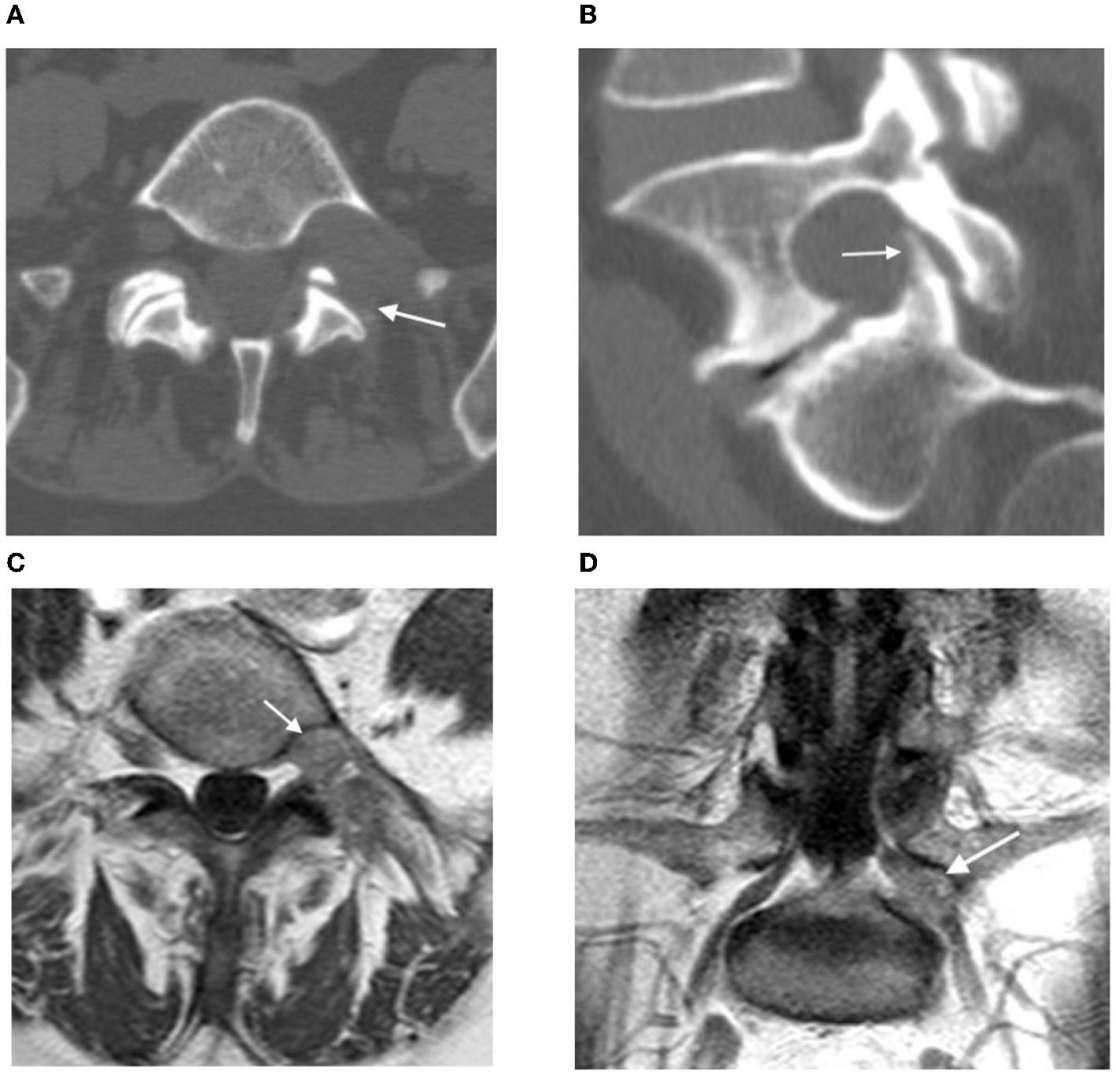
(A,B) Postoperative CT: the arrow shows the resection area of the left intervertebral joint L5-S1; (C,D) contrast-enhanced MRI 3 months after surgery: postoperative changes in the left L5 root (see arrow), no signs of contrast accumulation.
